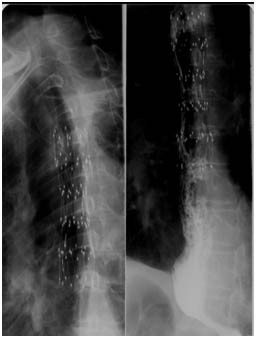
Chest X-ray showing expansion of the stent, 2 h after the procedure (left) and a barium swallow performed 12 h after the procedure, documenting complete sealing of a fistula (right).
